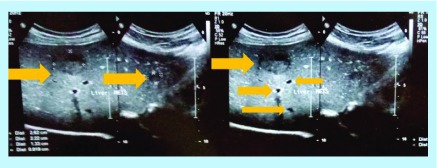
Ultrasonography showing the largest lesion in the liver 30 months after surgery for primary leiomyosarcomas.
